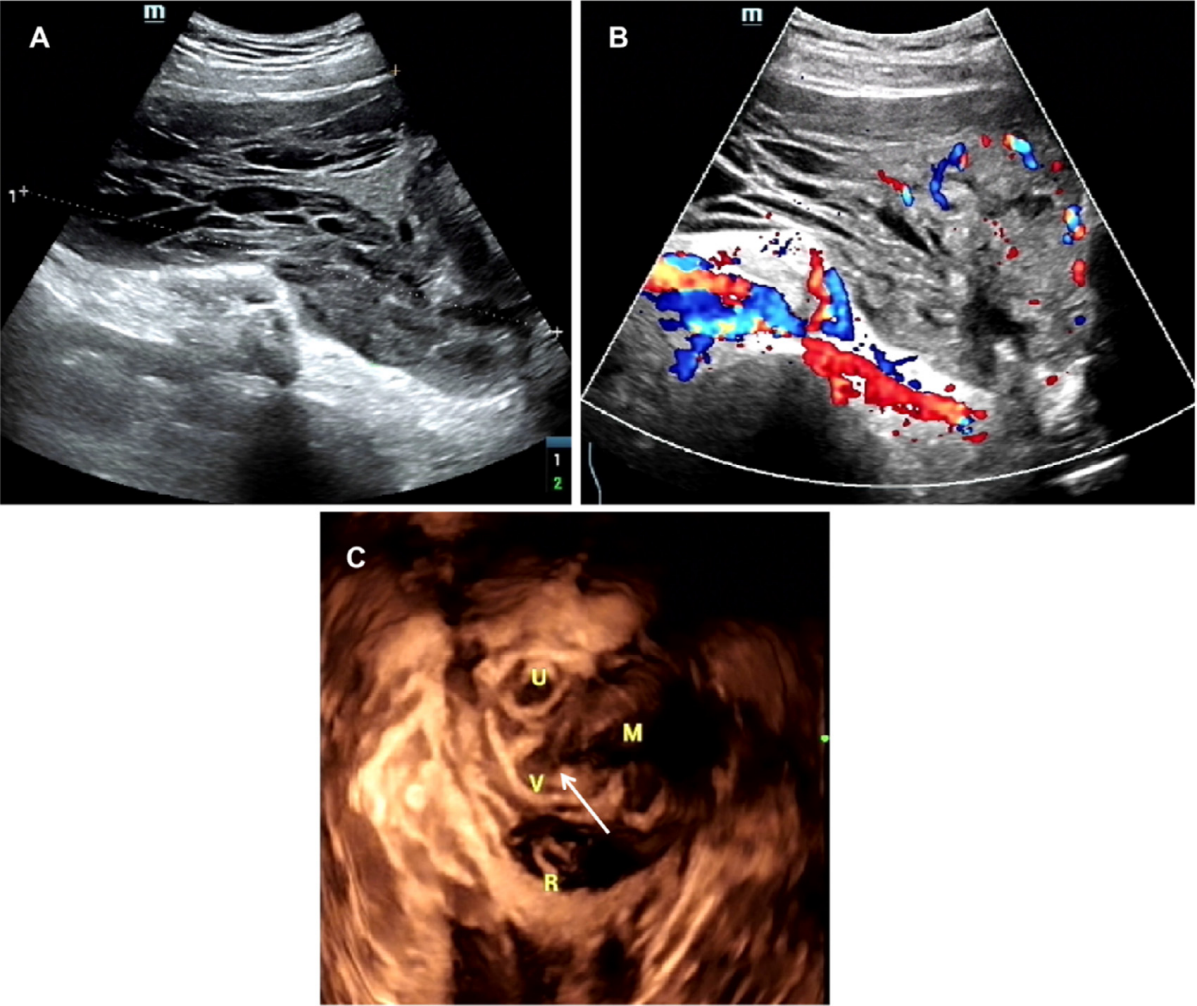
Ultrasonographic aspect of pelvic tumor and vaginal tumor. Abdominal ultrasound revealed a giant cystic-solid tumor (17 × 11.7 × 8.7 cm) in the pelvis, and (B) color Doppler flow imaging showed low blood flow in the tumor. (C) 3D ultrasound revealed a 3.5-cm vaginal defect (arrow) where pelvic tumor connected with vaginal tumor (3.6 × 6 cm). U, uterine; V, vaginal; M, mass; R, rectum.
